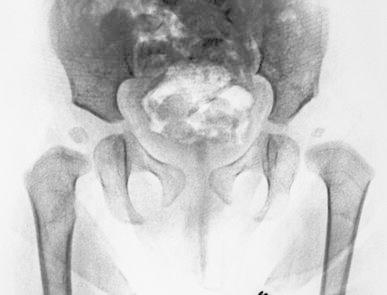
Inverted contrast anteroposterior pelvis radiograph demonstrating the common proximal femoral physis; *O’Brien’s Line*
